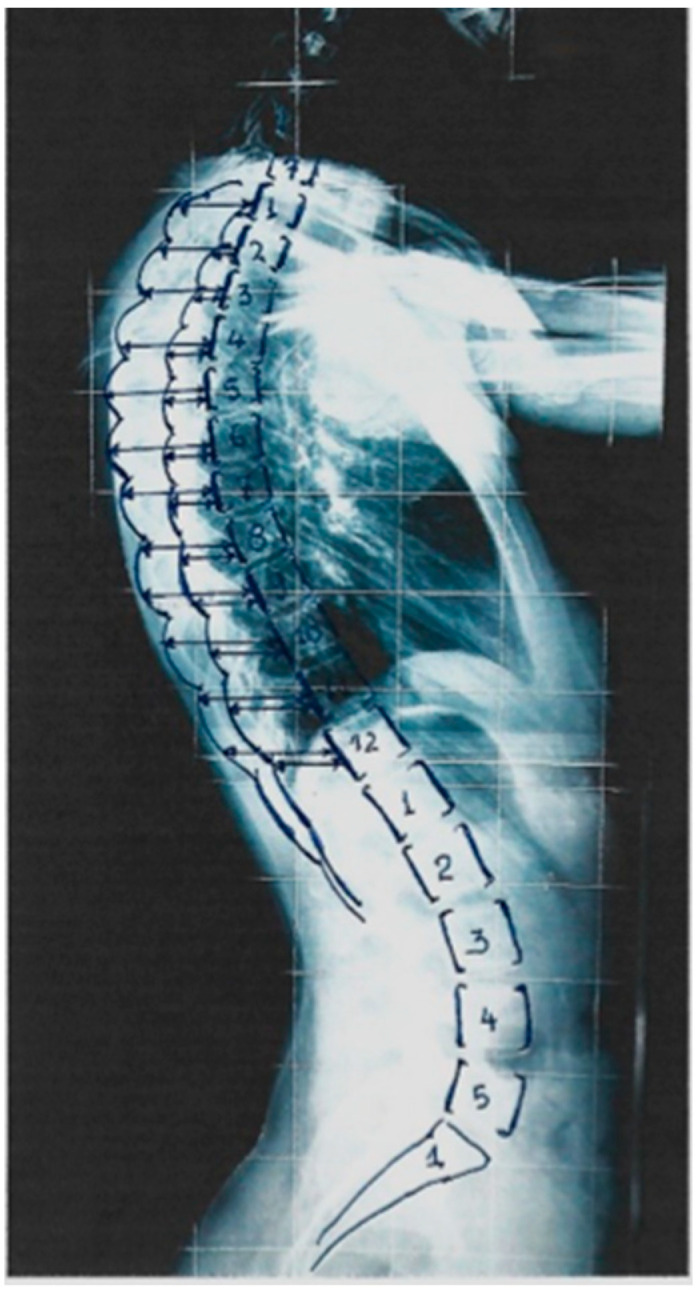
The way the segmental rib index (T1–T12) is assessed in the lateral spinal standing radiographs.
